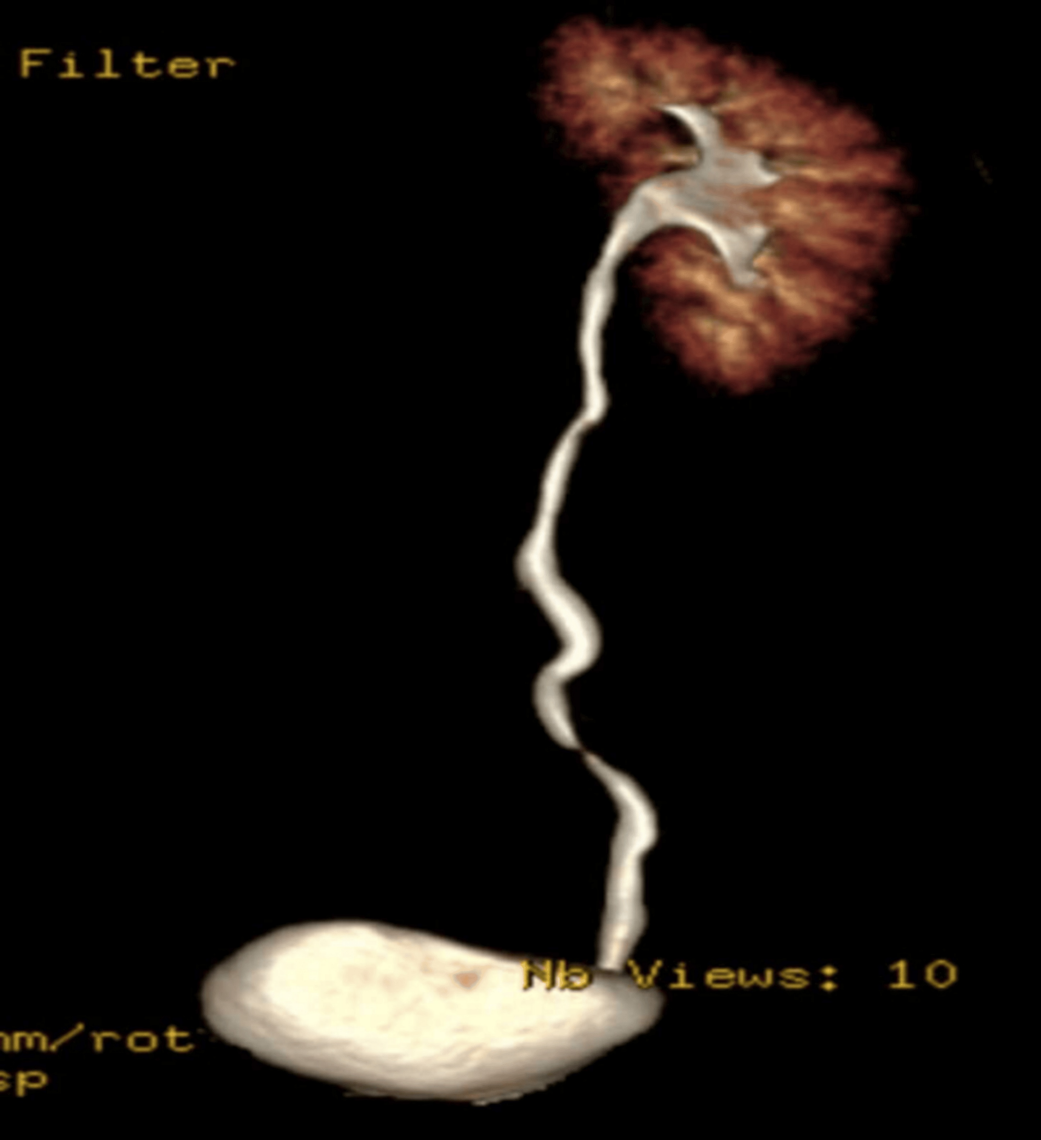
Coronal 3D CT urography image shows right renal agenesis. CT, Computed tomography
